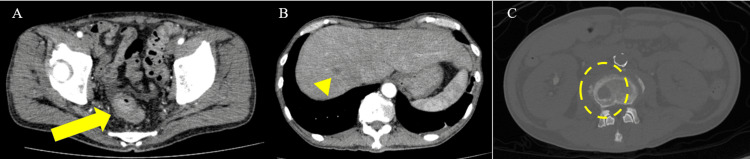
Abdominal computed tomography (A-C) Abdominal computed tomography shows an unevenly strengthened wall of the upper rectum (arrow) and liver (arrowhead) and bone (dashed circle) metastases
